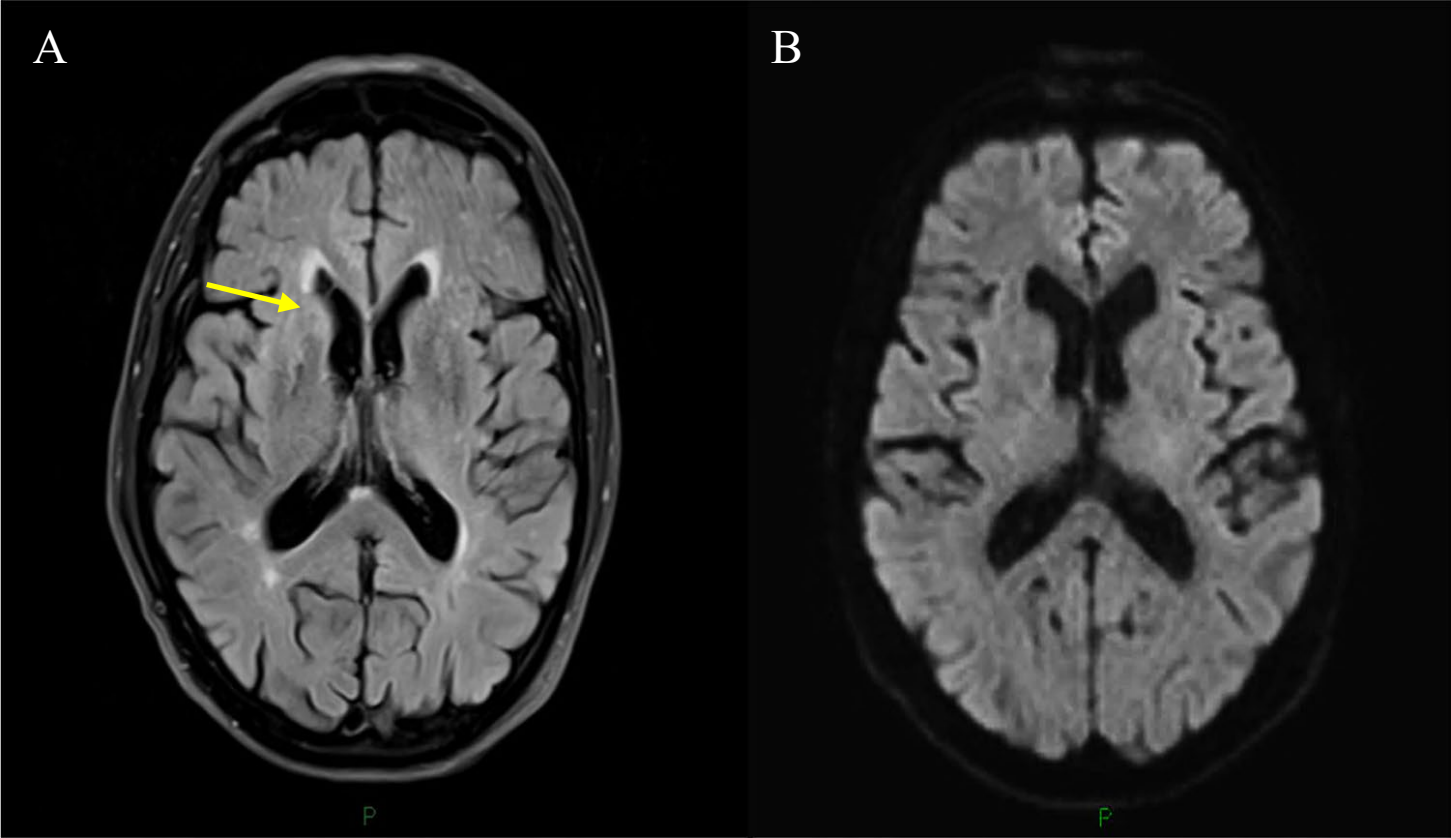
Axial MRI brain of a 74-year-old with autopsy confirmed Lewy body dementia. The FLAIR (A) shows subtle right caudate head signal abnormality with no associated high signal on DWI (B)
